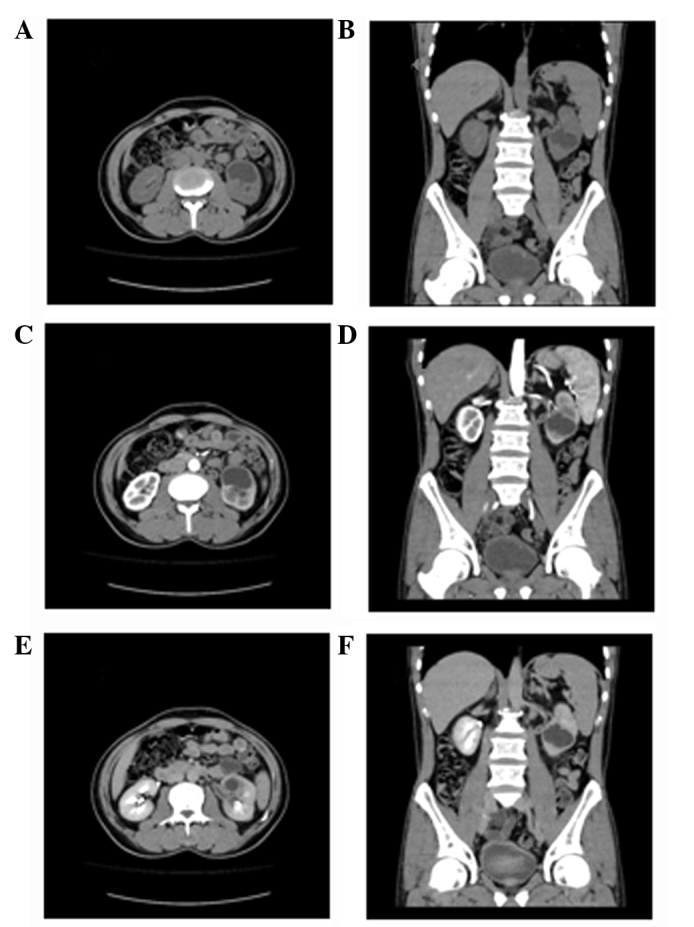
Case 2. CT scans show a low density focus (3.7×3.3cm) in the left kidney with a slight expansion of the pelvis, calices and ureter. (A and B) Plain scan; (C and D) arterial phase; and (E and F) delayed phase. CT, computed tomography.
